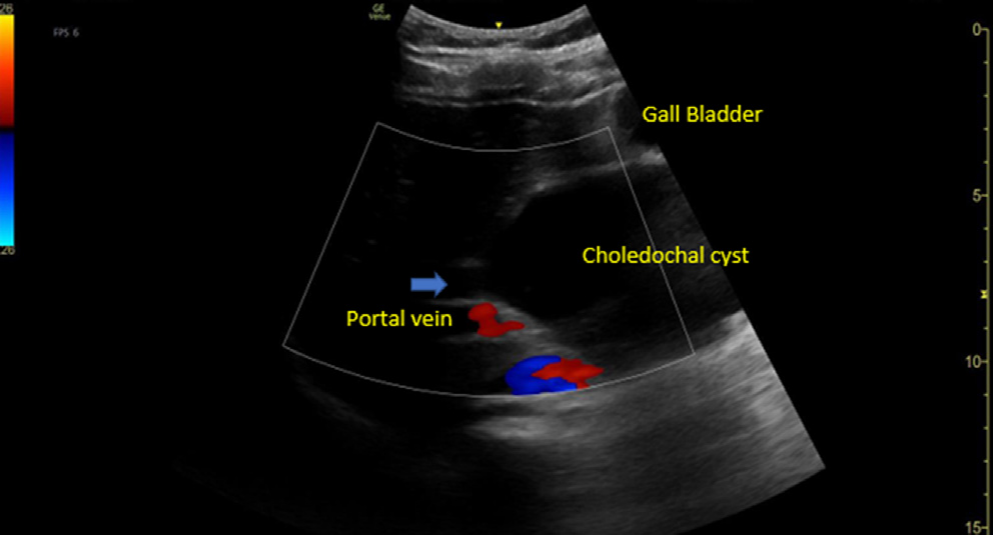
Point-of-care ultrasound showed an absence of color flow in the common bile duct (blue arrow) and choledochal cyst.
